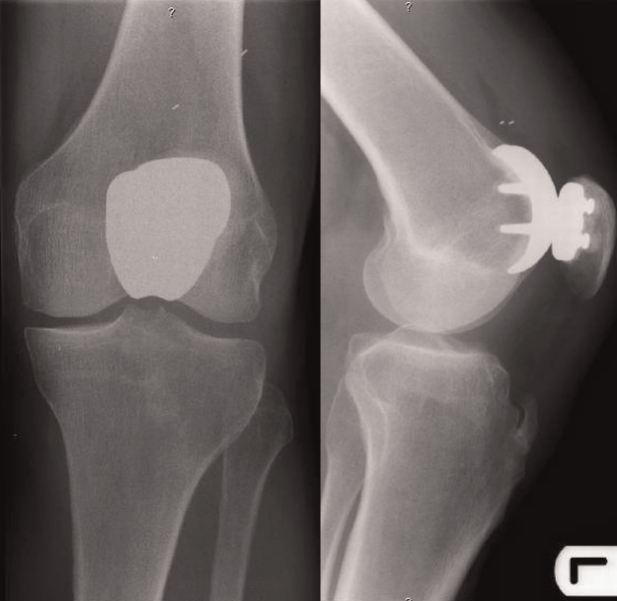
Radiograph of left knee showing dissociation of the polyethylene patellar component. The suprapatellar position of the polyethylene is demonstrated by the two metal markers visible just proximal to the trochlear component.
